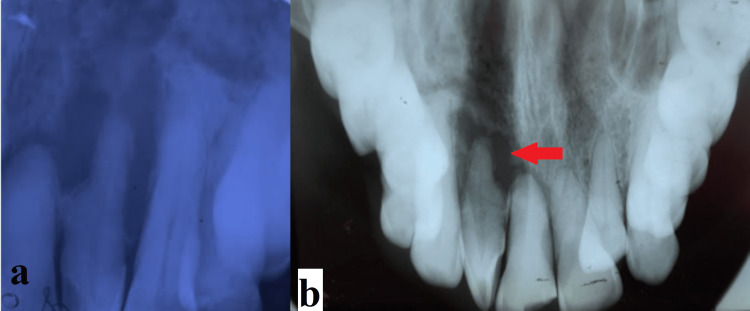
Intraoral periapical radiograph and occlusal radiograph. a: Intraoral periapical radiograph showing ill-defined periapical radiolucency with bone loss in relation to the 21, 22, 23 region. b: Occlusal radiograph revealing mixed radiopaque and radiolucent area superior to periapical radiolucency with altered trabeculae pattern in relation to the 21, 22, 23 region (red arrow).
